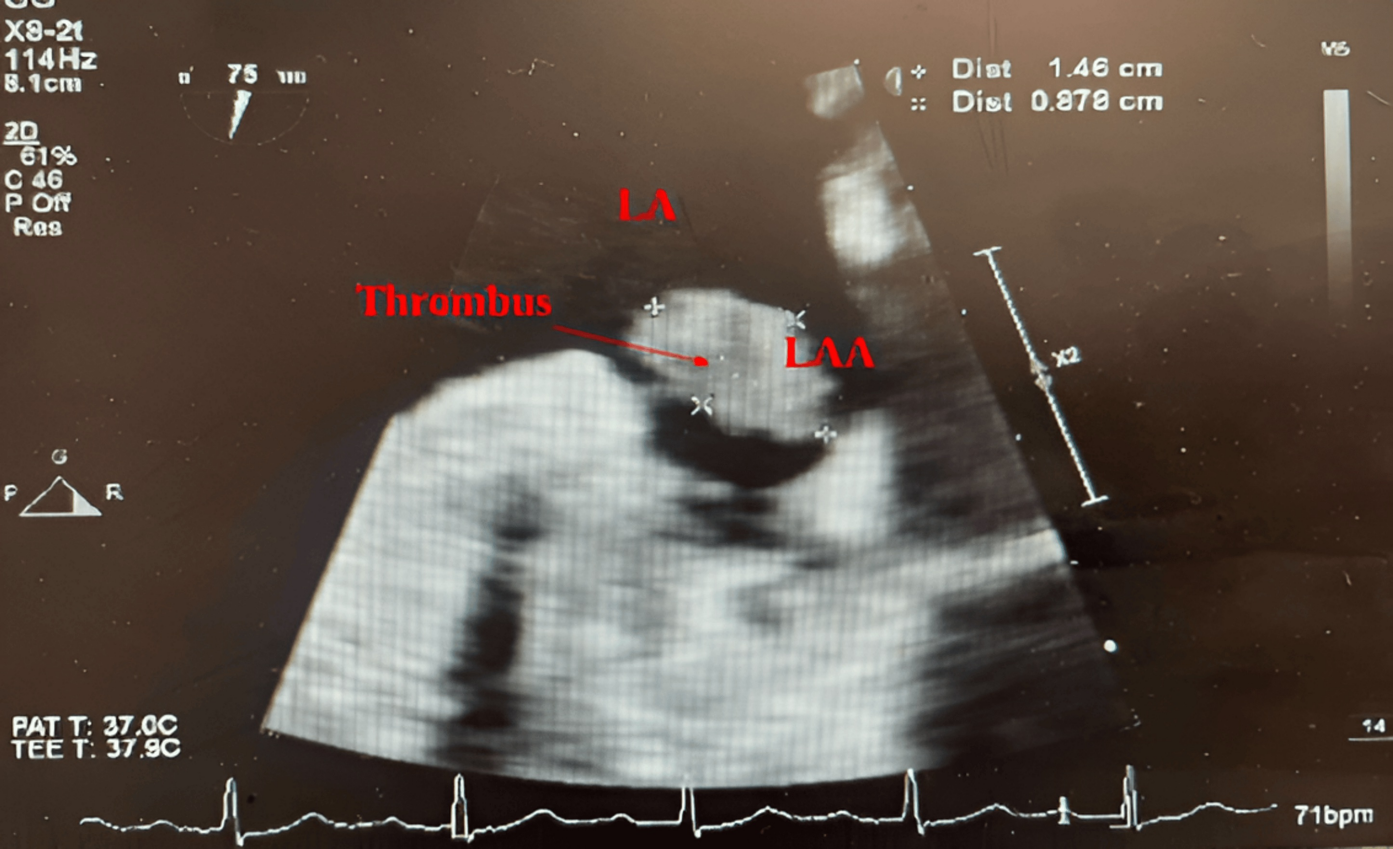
Transesophageal echocardiogram in 2D mode showing a large, mobile thrombus in the left atrial appendage, measuring 8 x 15 mm. The thrombus appears as a hyperechoic mass with defined borders.
